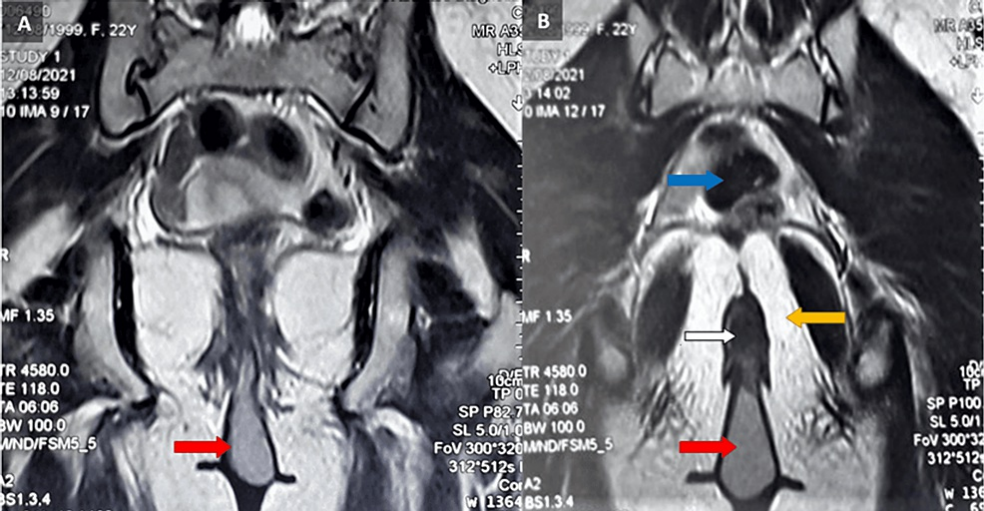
Axial MRI scan of the tailgut cyst. This MRI scan shows a hyperintense lobulated soft tissue lesion (red arrow) in the region of the anal canal (white arrow). It arises from the external anal sphincter on the left side at the 4 o'clock position. It bulges into the anal canal and laterally has a mild mass effect on the left ischiorectal fossa (yellow arrow). Also shown for reference is the sigmoid colon (blue arrow).
